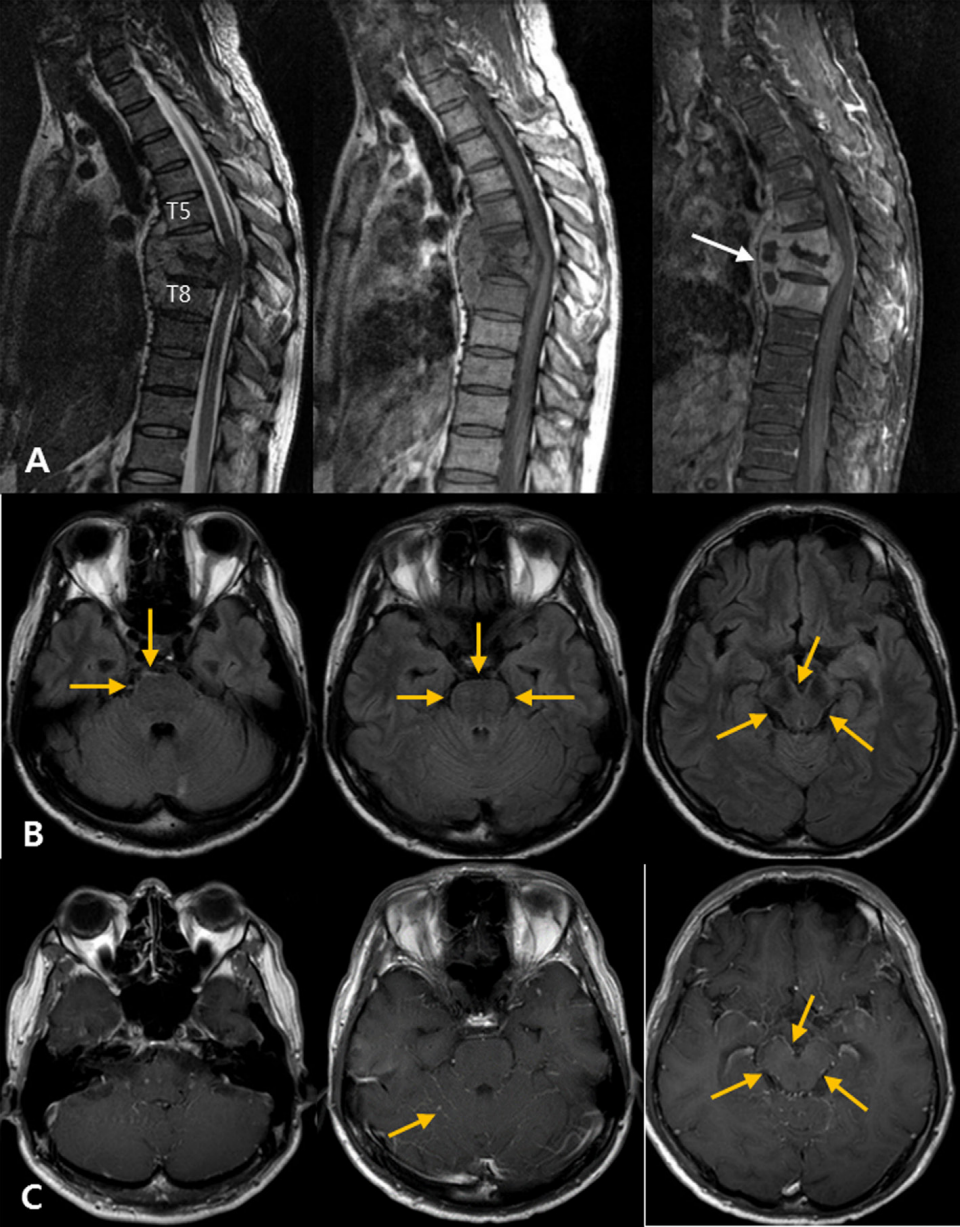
Atypical tuberculous meningitis in a 45-year-old man with low back and radiating pain. (A) Spine magnetic resonance (MR) shows subligamentous spreading pattern of spondylitis with T6–7 endplate erosion and paravertebral abscess formation (arrows), suggestive of tuberculous spondylitis. (B) One month later, the patient became mentally confused. Post-contrast T1-weighted MR images show linear high signal intensities along the pial surface of the infratentorium, including the cerebellar folia and surface of the brainstem. (C) Matching fluid-attenuated inversion recovery images also show subtle abnormal high signal changes along the pial surface of the infratentorial structures.
